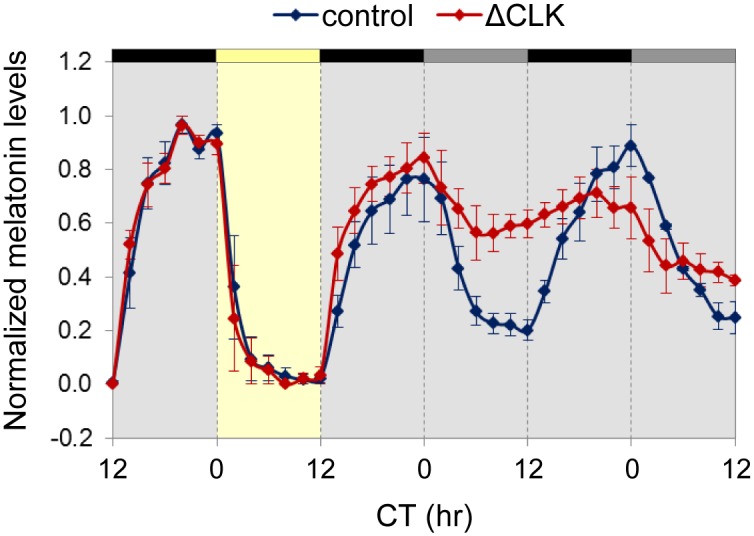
Clock-controlled rhythms of melatonin production are disturbed in ΔCLK-expressing pineal glands. Circadian rhythms of melatonin release from cultured pineal glands of Tg(*aanat2*:EGFP-ΔCLK) adult fish are disturbed under DD (*p*<0.05, Kolmogorov-Smirnov test). Yellow bar represents the light period, black bars represent dark, and gray bars represent dark during subjective day. Values indicate the normalized amount of melatonin produced by the glands. Error bars represent SE (*n* = 3). CT, circadian time.
